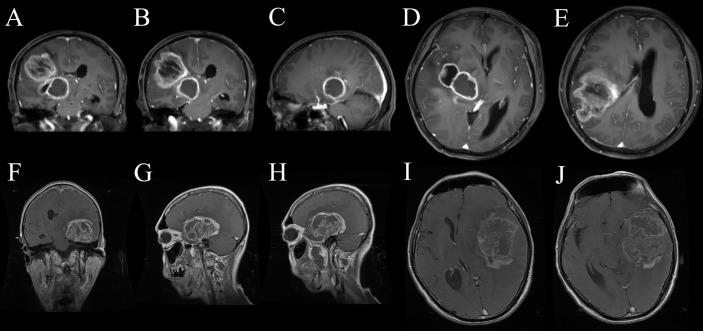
The appearances of ring enhancement on enhanced MRI of 1 GBM patient with MUT BRAF (A–E) and another one with WT BRAF (F–J). The patient with MUT BRAF showed almost all the characteristic radiological appearances we proposed such as multiple ring enhancement appearances (A), multiple lobes (B), regular shape (C), uniform thickness of ring (D) and small diameter of ring (E). The patient with WT BRAF showed the opposite images such as single ring enhancement appearances (F), single lobes (G), irregular shape (H), nonuniform thickness of ring (I) and relatively larger diameter of ring (J).
